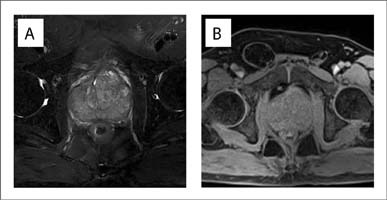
Irregular prostatic mass showing an isointense signal on T1-weighted images (A) and T2-weighted images (B), with an obscure boundary.
